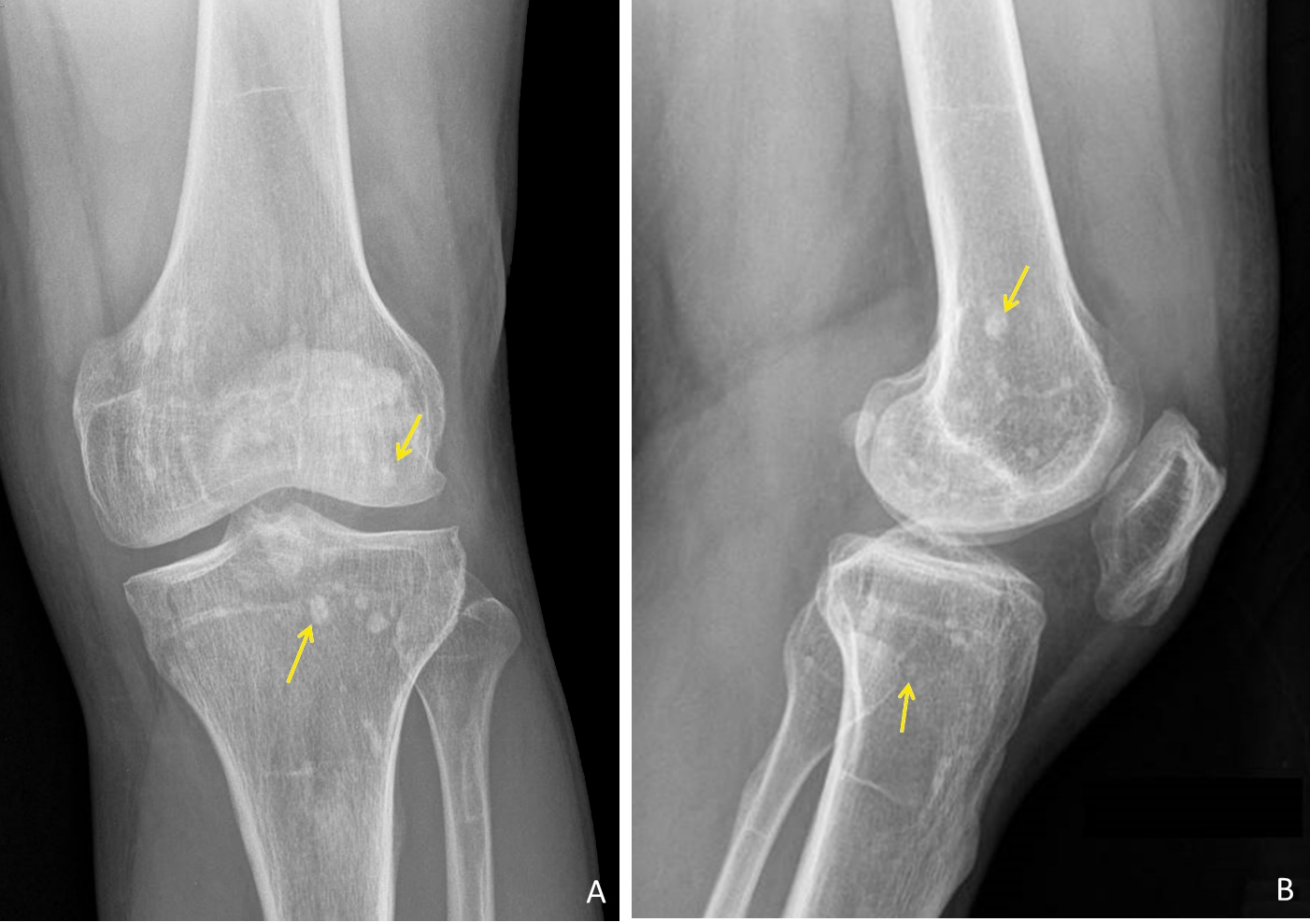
Radiographs of the Left Knee. Anteroposterior (A) and lateral (B) radiographs of the left knee demonstrating multiple non-aggressive appearing, sclerotic foci (yellow arrows) in the distal femoral and proximal tibial metaphysis and epiphysis, as well as in the proximal fibular metaphysis.
